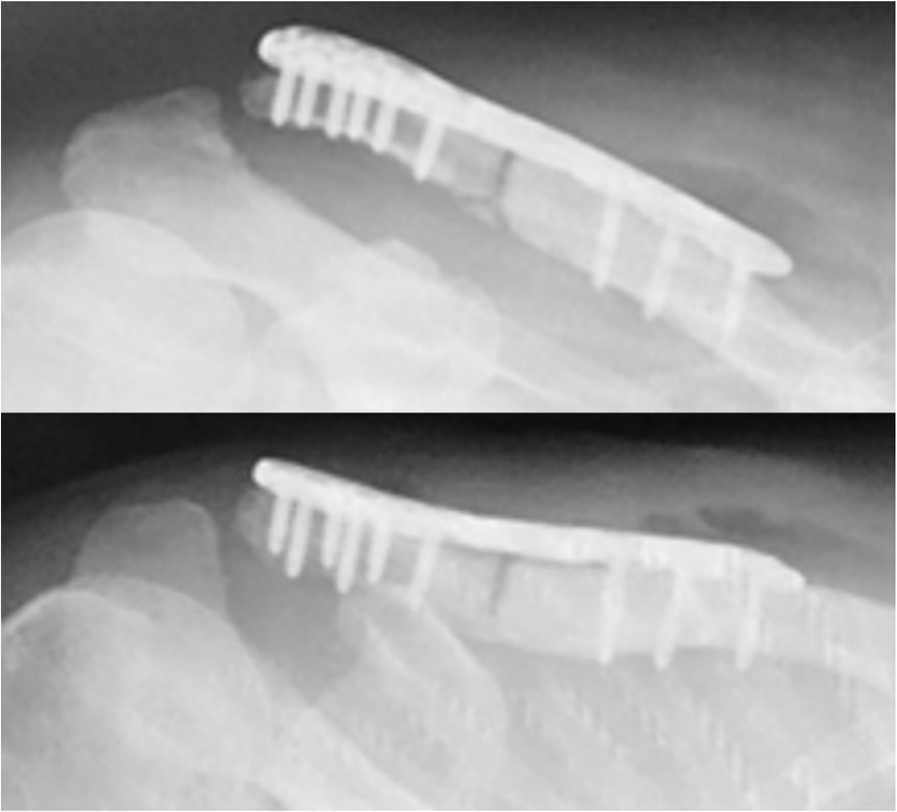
Intraoperative x-ray radiography images from the present surgery. X-ray radiography images after open reduction and internal fixation of the right clavicle revealed that the clavicle fracture was reduced and fixed by a plate
